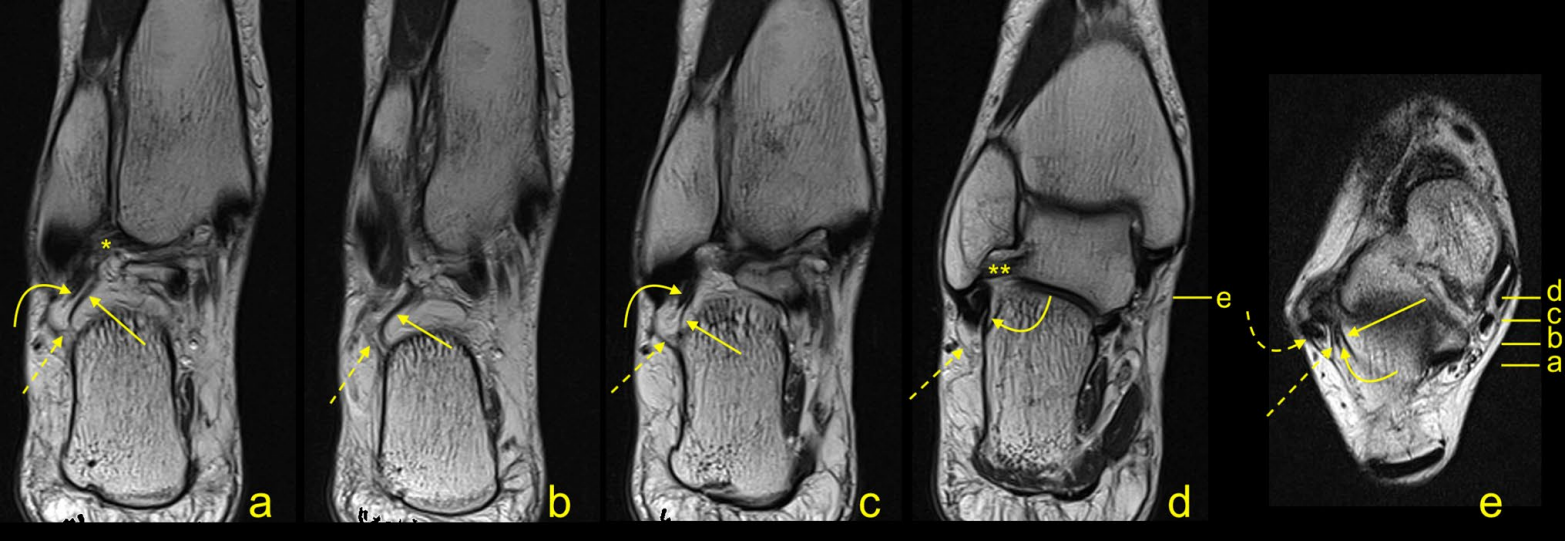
A 43-yeard-old patient with suspicion of a tumor. The MRI did not reveal a tumor in the soft tissue. The oblique sections (a–d) and the axial cross-section (e). Levels of cross-sections are labeled on figures (d,e). Connections of the CFL (curved arrow) with the FTCL (straight arrow), the PTS (dashed arrow), the superior peroneal retinaculum (dashed curved arrow), the PTFL (*), and the ATFL ().
